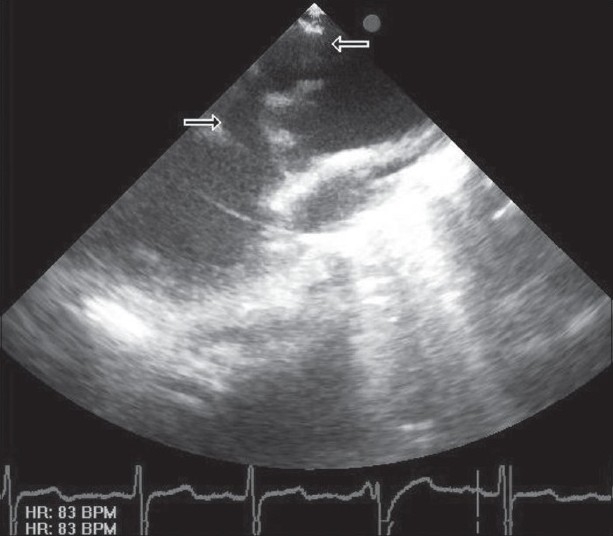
Two-dimensional imaging of the tunnel in transthoracic echocardiographic examination
